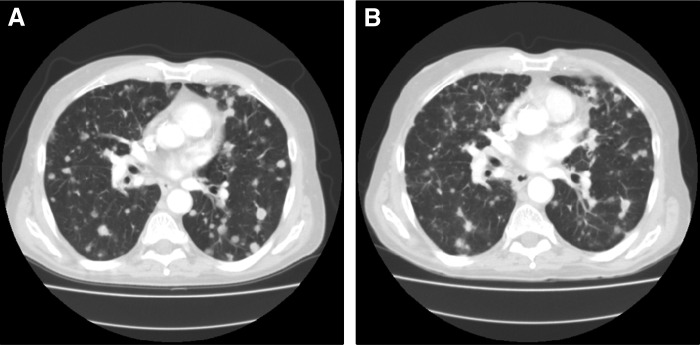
Chest computed tomography scans from January 2005 (A) and April 2015 (B) demonstrating interval improvement in lesions following initiation of trastuzumab and pertuzumab.
